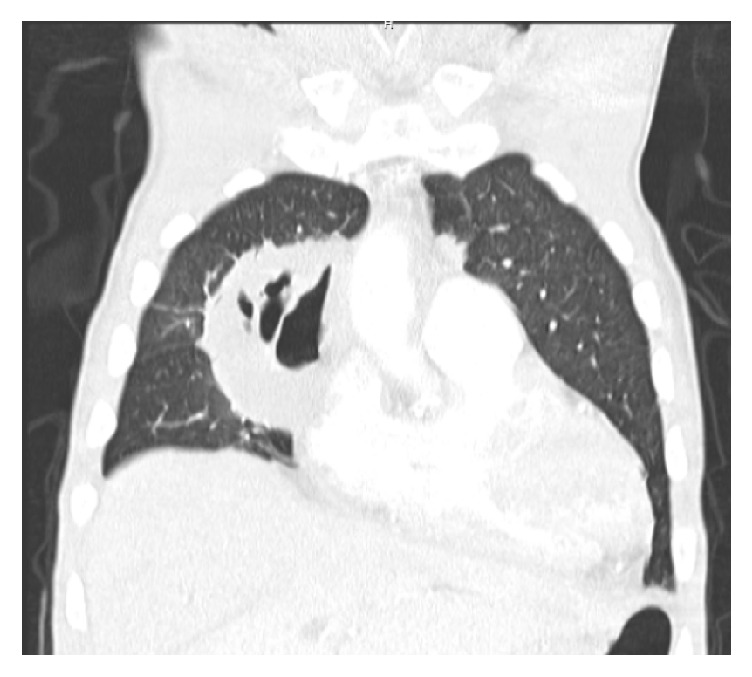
CT of the chest revealing a large right middle lobe cavitary mass (6.5*∗*7.6*∗*8.1 cm^3^) with bilateral pulmonary nodules and a small pericardial effusion.
